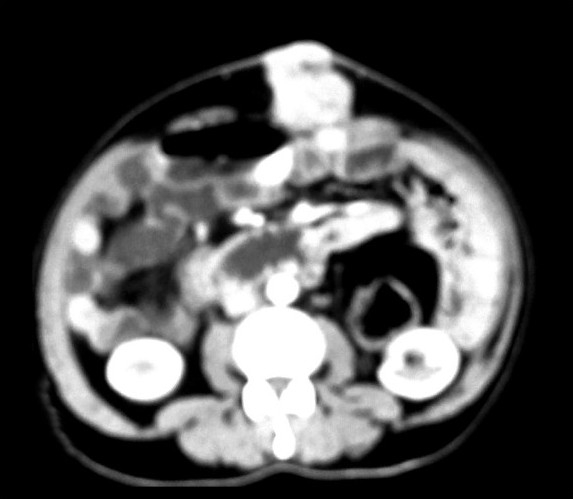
CT scan showing parietal mass in anterior abdominal wall
